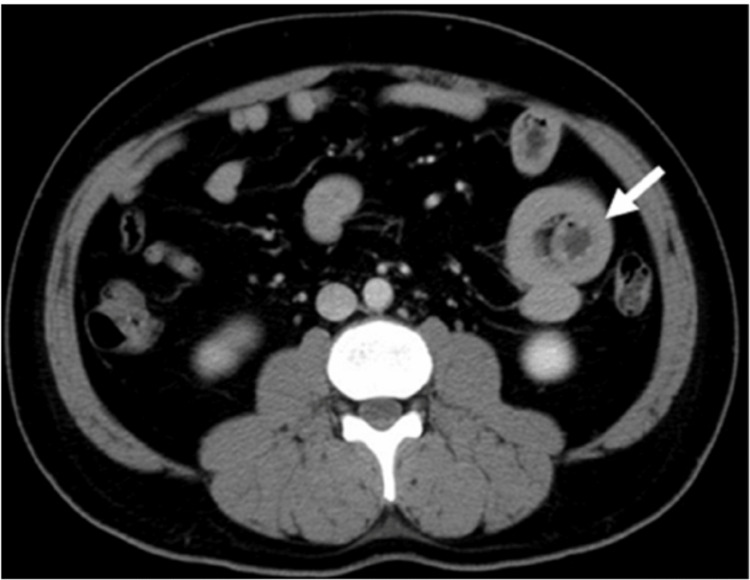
A CT scan of the abdomen showed a 7-cm mass in the jejunum with associated bowel wall thickening (arrow).
